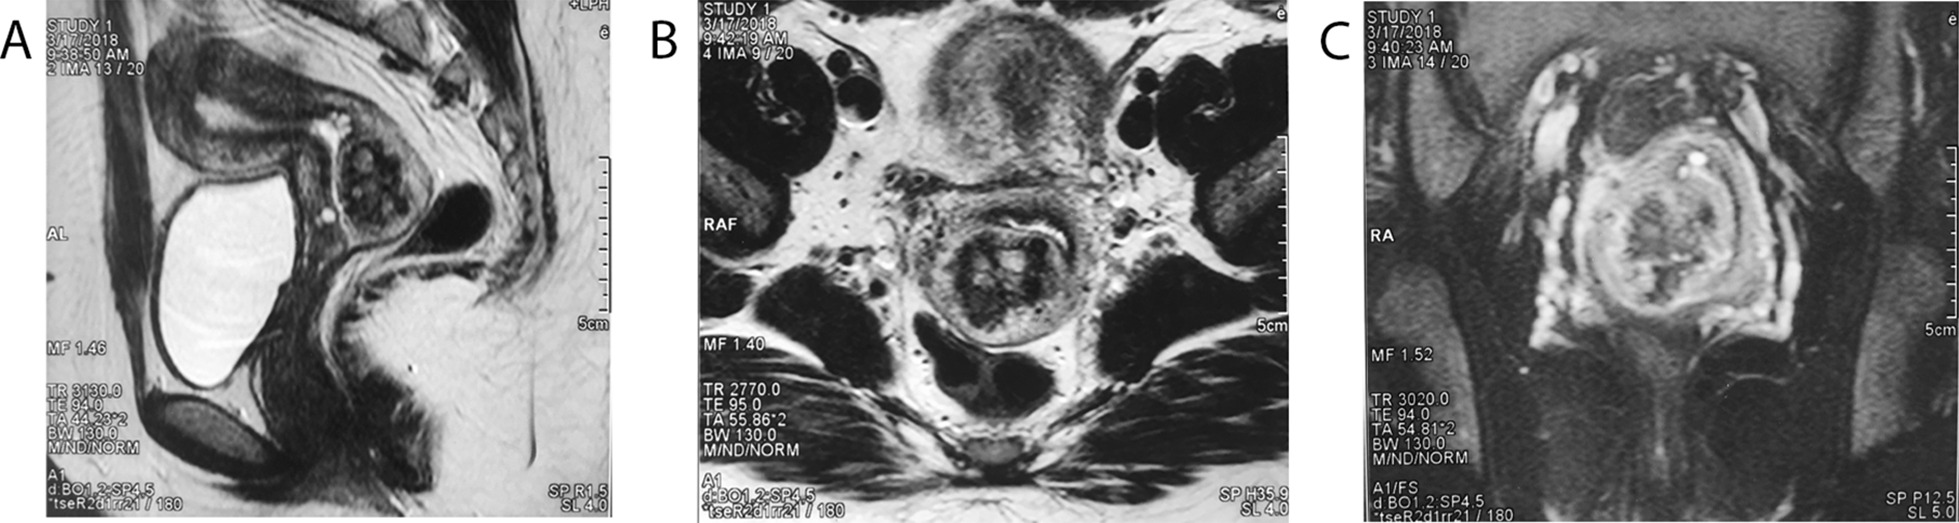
Magnetic resonance imaging of a 22-year- old woman with choriocarcinoma. Sagittal T2-weighted image (a) and axial T2-weighted image (b) demonstrate a heterogeneous, hyperintense, focal mass located in the posterior cervical lip. Coronal (c) contrast-enhanced T1-weighted images show heterogeneous enhancement of the tumor. Tortuous flow voids consistent with vessels can be observed in the parametrium, indicating tumor hypervascularity
